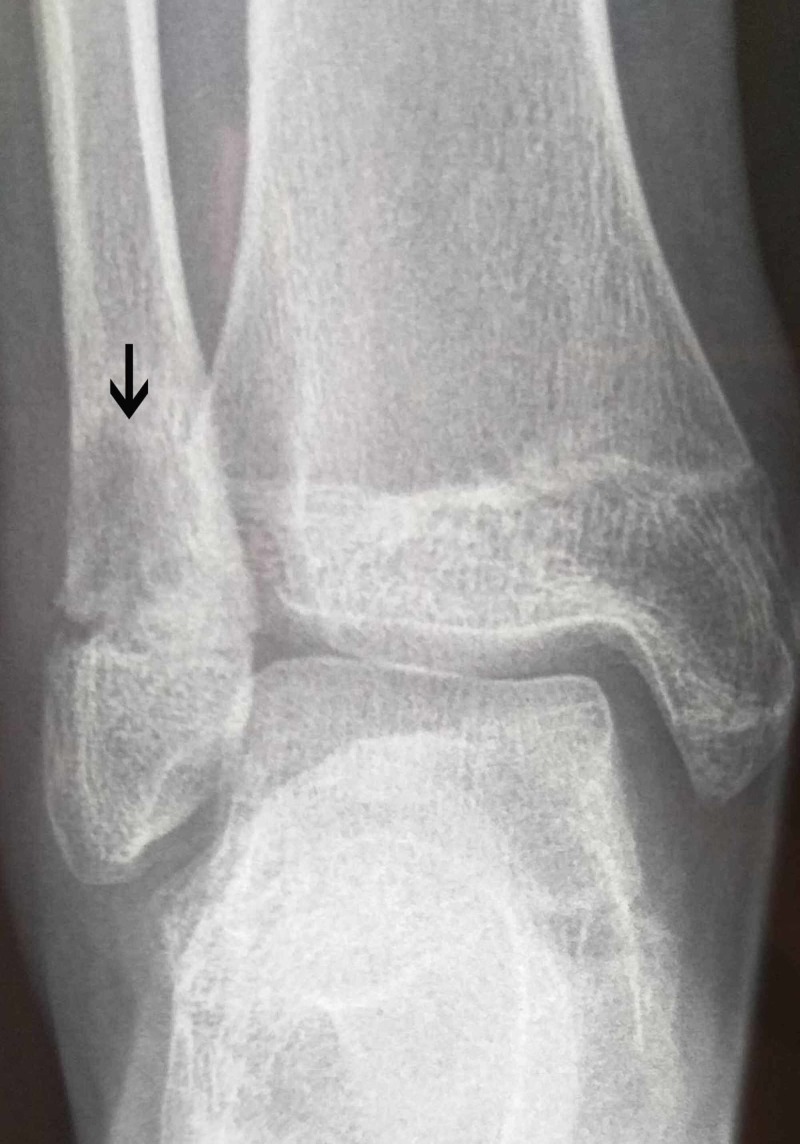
X-ray of the right ankle Three months after the biopsy the radiological image was improved. The osteolytic changes of the right fibula were less evident (black arrow)
